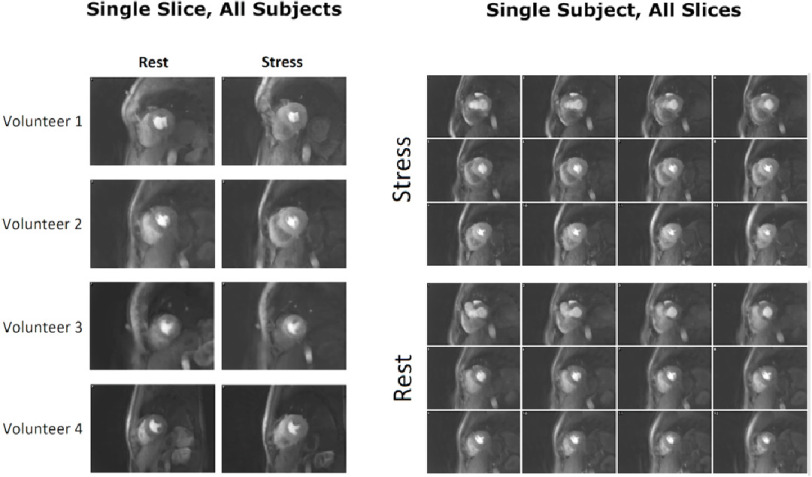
Healthy volunteer overview. An overview of the four healthy volunteer subjects, demonstrating a single central slice from 3D-SOS-FPP at a single time point of GBCA arrival in the left ventricle for each subject (left), and for 12 reconstructed five mm slices from base to apex of a single temporal frame (right). The remaining 4 slices are not shown as those are at the edges of the slab selection and partition-encoding direction as is usual in 3D MRI. All of the images from a 3D-SOS-FPP shot are at the same cardiac timing (see Discussion) unlike the multiple slices of 2D-FPP.
